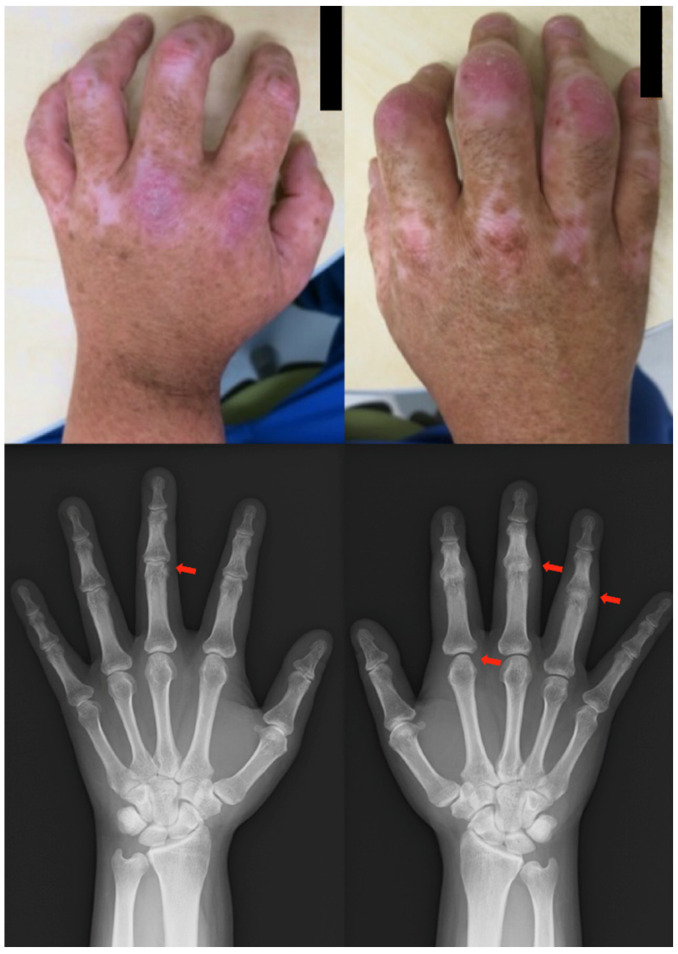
Clinical photographs and radiographs of the hands in case 6. Radiographs show bone erosion, loss of cleft space, and joint destruction in the PIP joint of the hands (red arrow). PIP, peripheral interphalangeal.
